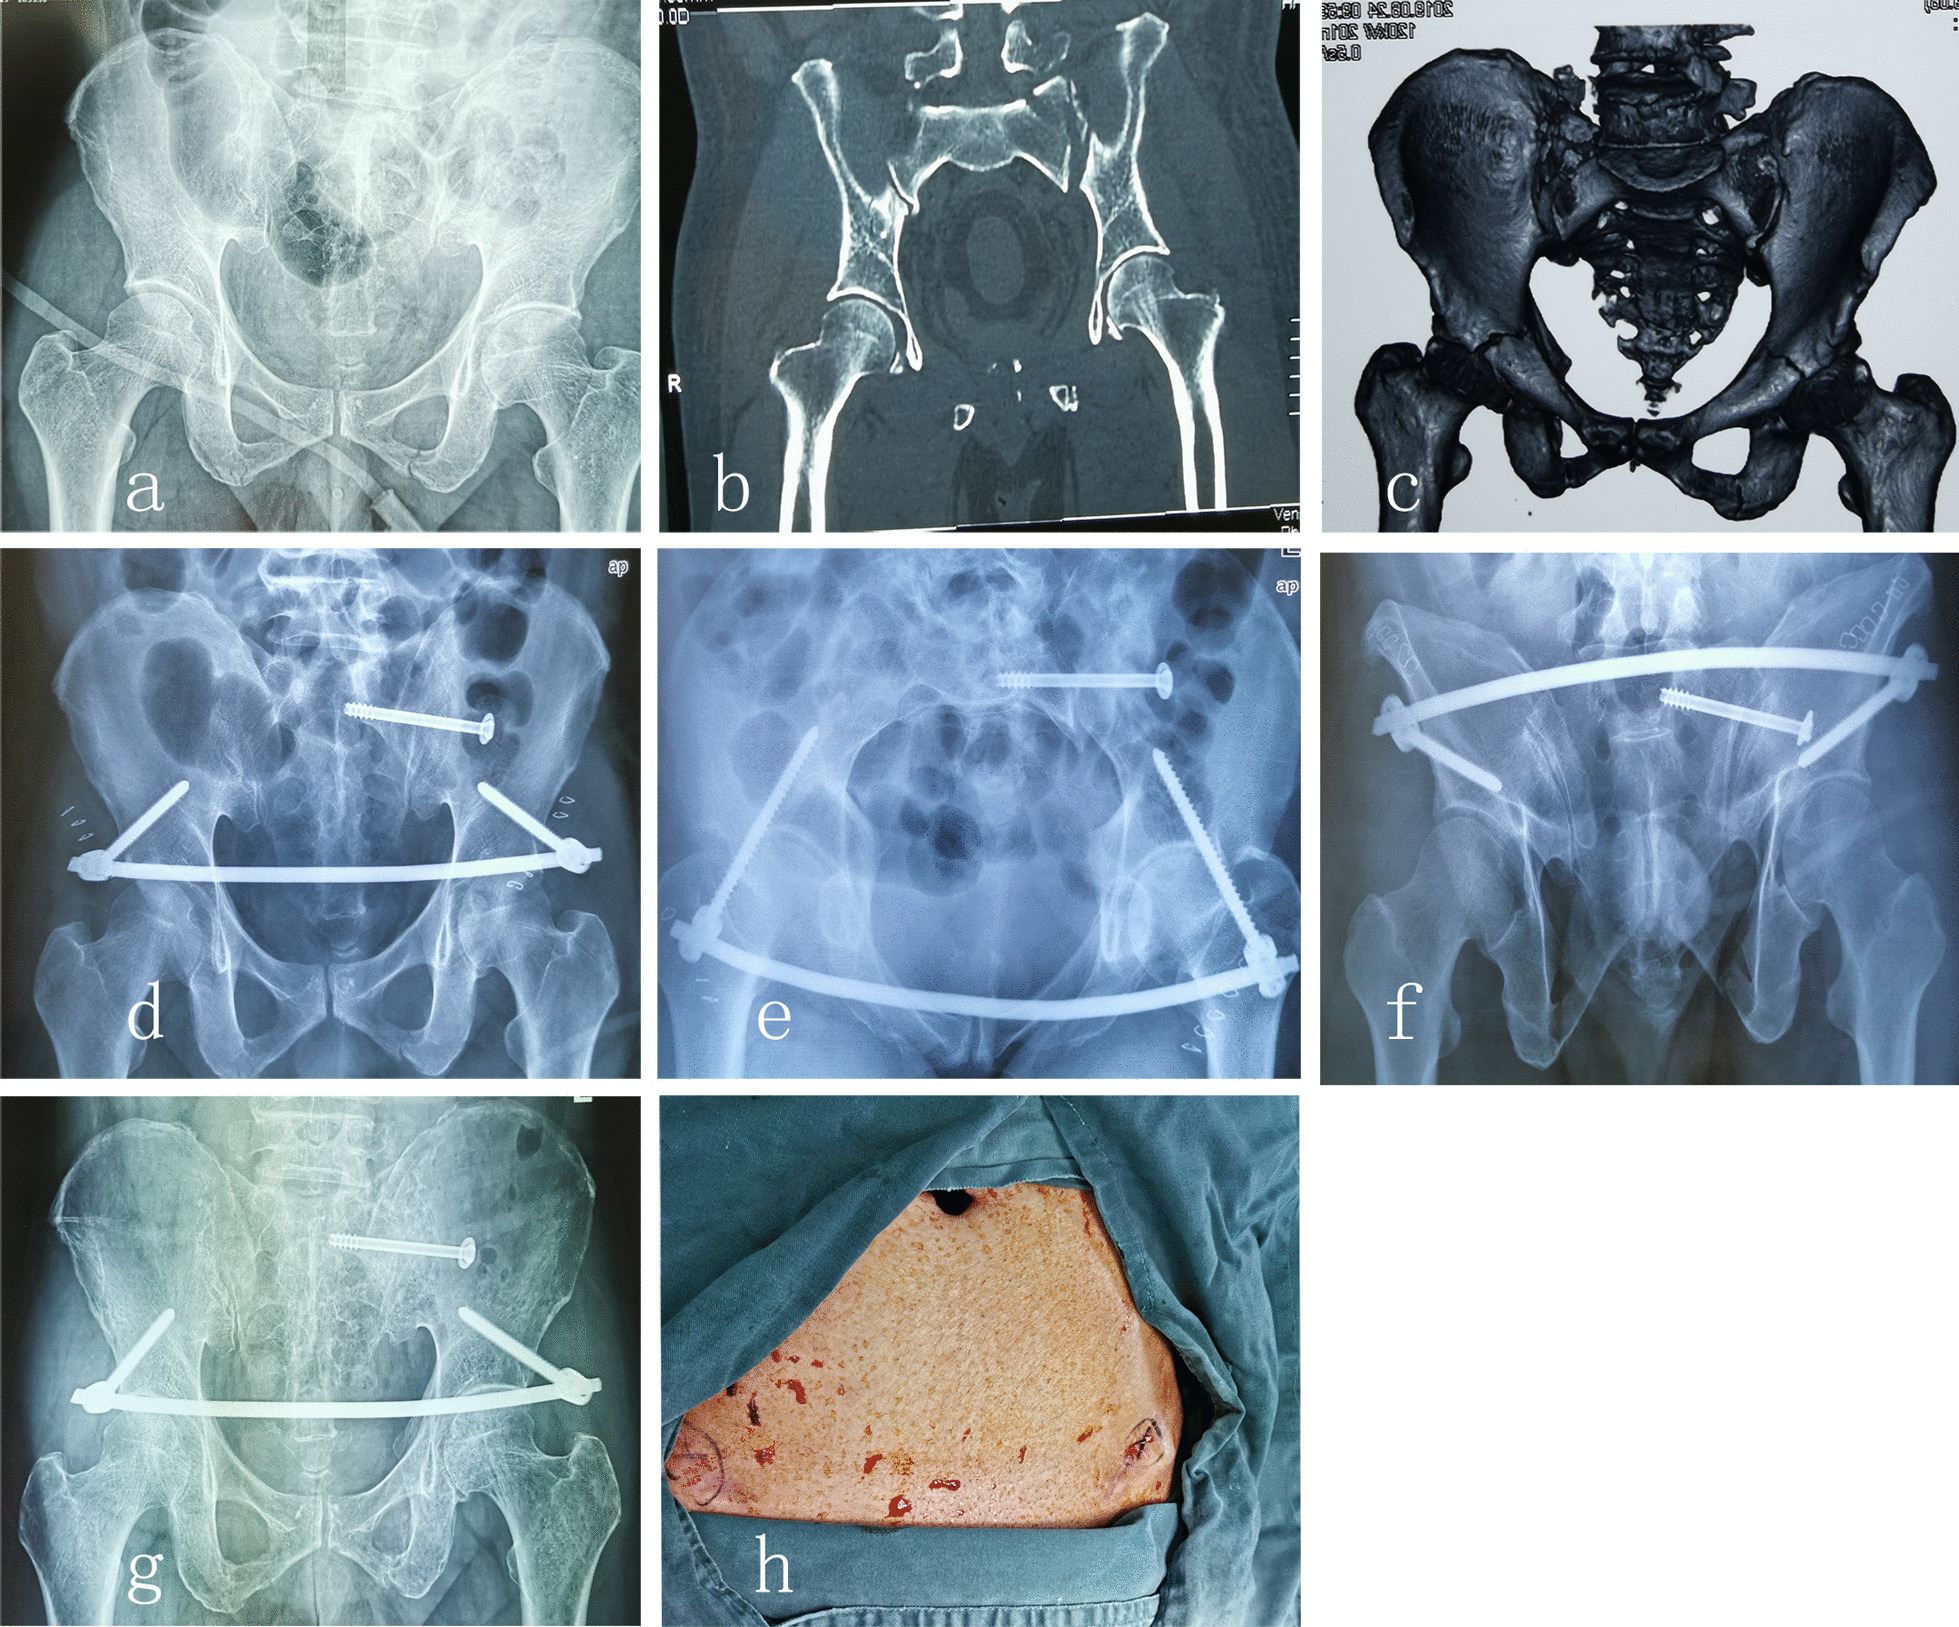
A series of images depicting the clinical course of a patient (male, 57 years, AO/OTA type C1) who developed loosening of screws at 6 months post-surgery. Preoperative images of the pelvis, including anteroposterior (a) and coronal (b) radiographs, and three-dimensional CT scan images (c), all depicting left sacroiliac joint fracture dislocation with complete instability, and anterior pelvis fracture with concomitant bilateral superior and inferior pubic rami fractures. Plain radiographs taken 1 day after INFIX surgery, showing anteroposterior (d), inlet (e), and outlet (f) views of the pelvis. Images taken 6 months after INFIX surgery, showing screw loosening on anteroposterior radiographs (g) and compression of the skin (h)
